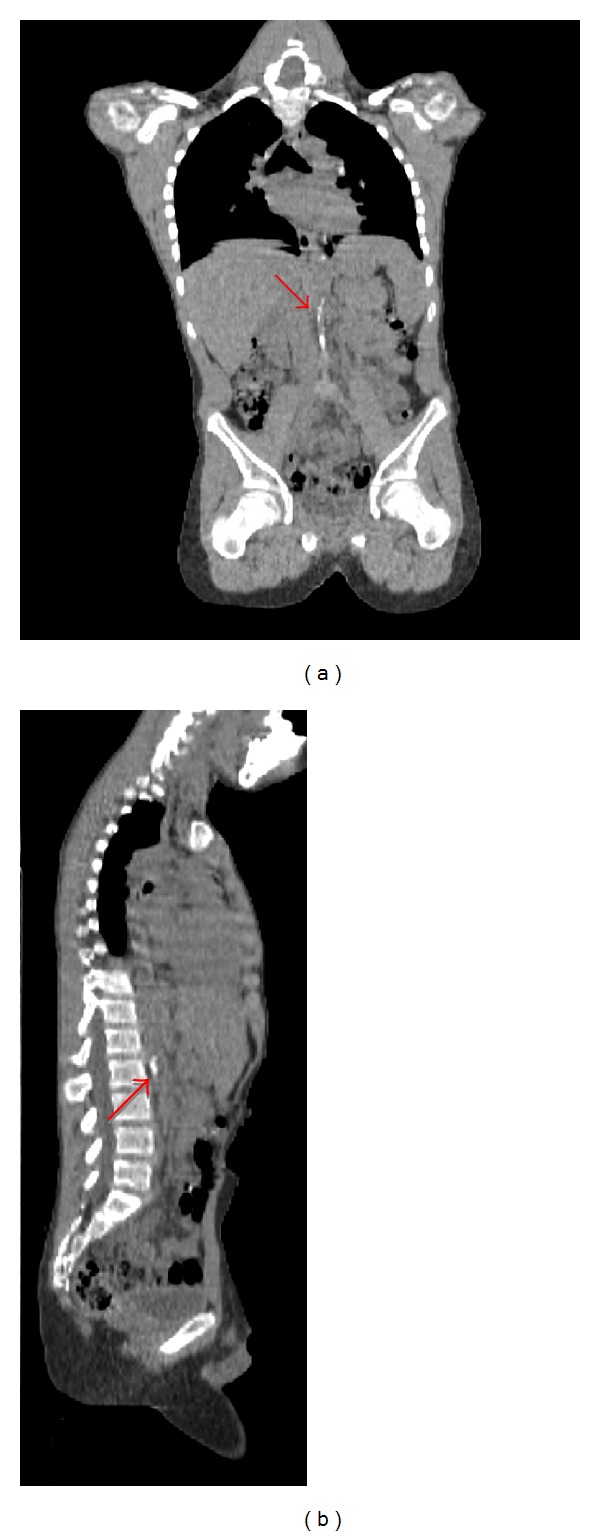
An unenhanced computerized tomography (CT) image (coronal and sagittal reformatted) showing aortic calcification (arrow).
